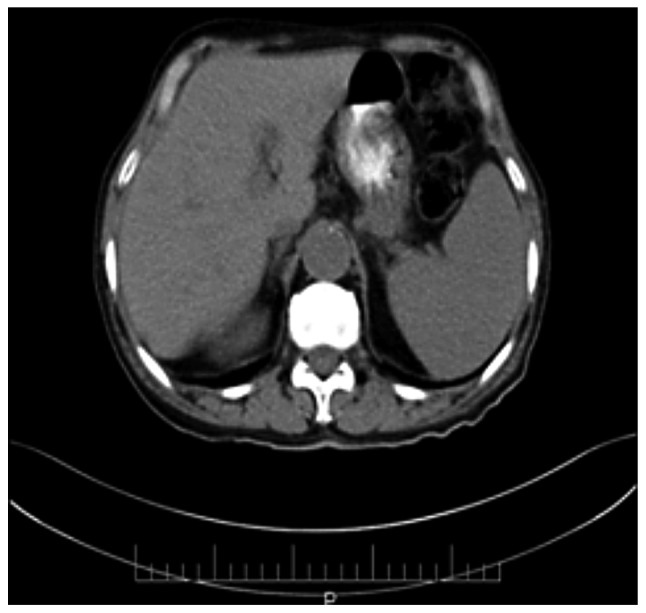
Computed tomography scan revealing a gastrointestinal stromal tumor of the stomach measuring 6×4×5 cm.
